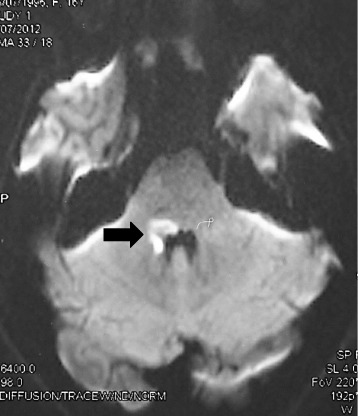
Diffusion-weighted magnetic resonance imaging showing restricted diffusion in the right middle cerebellar peduncle (arrow). The lesion extended from the pons to the posterior spinal cord (not shown).
